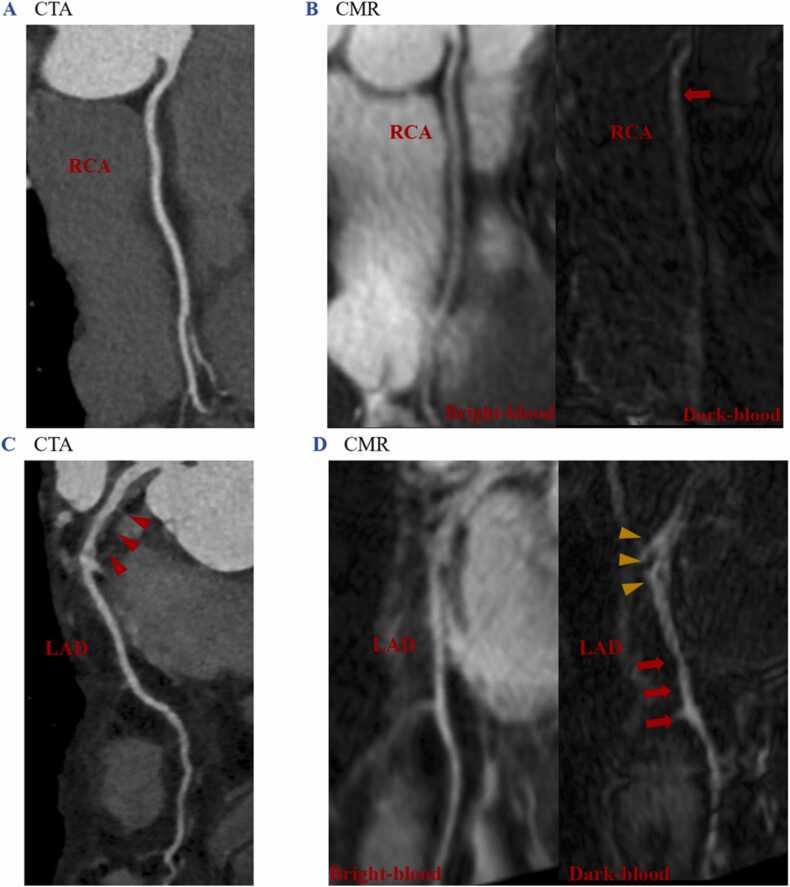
CTA and CMR images of IgG4-RD patients with coronary involvement. A 56-year-old female IgG4-RD patient without any cardiovascular symptoms, the CTA showed no abnormal changes (A). CMR bright-blood image showed similar ability to manifest the anatomical structure of the coronary lumen as CTA, but the accordingly dark-blood image could manifest CE in the proximal RCA wall (red arrow), suggesting coronary involvement (B). A 50-year-old male patient with IgG4-RD experienced occasional palpitations and shortness of breath. CTA indicated mild-to-moderate thickening of the proximal LAD wall (red arrowheads) (C). However, the CMR dark-blood image revealed diffuse CE in the LAD wall, involving both thickened (yellow arrowheads) and unthickened (red arrows) segments (D). *IgG4-RD* IgG4-related disease, *CTA* computed tomography angiography, *CMR* cardiovascular magnetic resonance, *RCA* right coronary artery, *LAD* left anterior descending.
